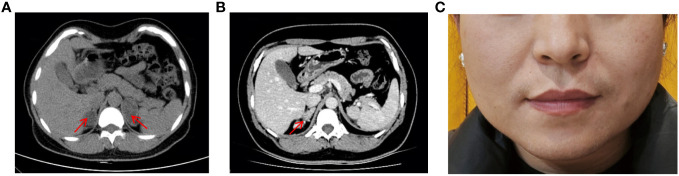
Masculine features and Abdominal CT scan of patient. (A) Abdominal CT scan showed bilateral adrenal hyperplasia, with a nodule on the right approximately 27×22mm and a nodule on the left approximately 42×28mm. (B) Abdominal CT scan revealed recurrence of the right adrenal gland hyperplasia two years after surgery. (C) Physical examination revealed greasy skin pigmentation, facial acne, and slight mustache on the upper lip.
